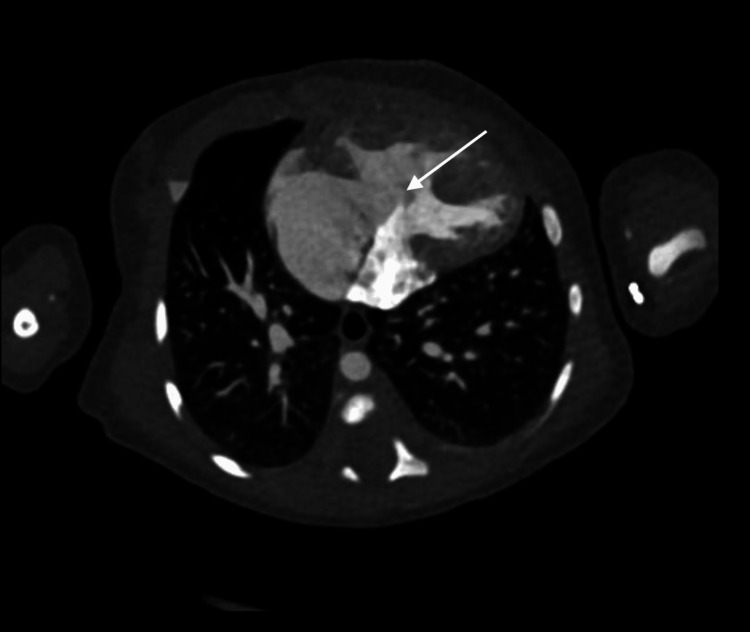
Axial contrast-enhanced computed tomography image demonstrating a complete, unbalanced atrioventricular septal defect with right ventricular dominance The white arrow highlights the common atrioventricular junction overriding a hypoplastic left ventricle, consistent with right ventricular dominance.
